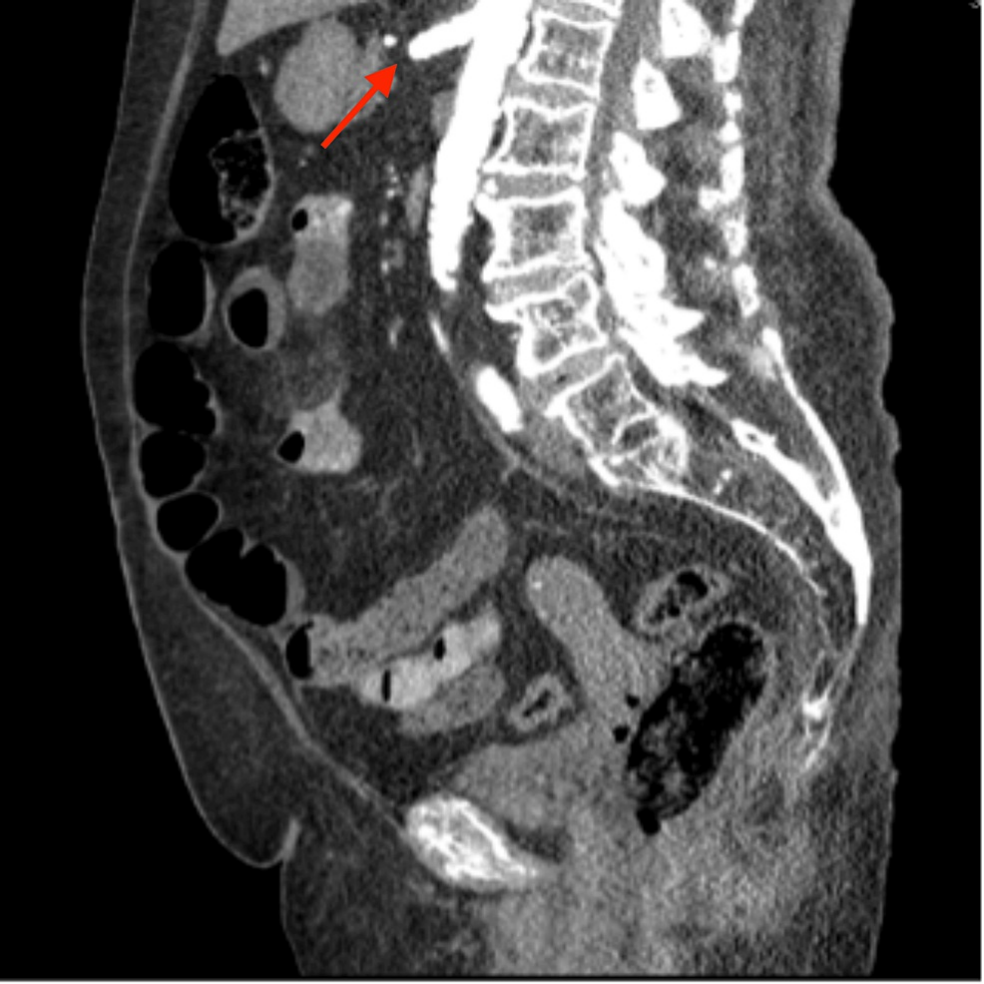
The CTA shows the CA and the SMA arising from a common trunk (red arrow) CTA: CT angiogram, CA: Celiac artery, SMA: Superior mesenteric artery
